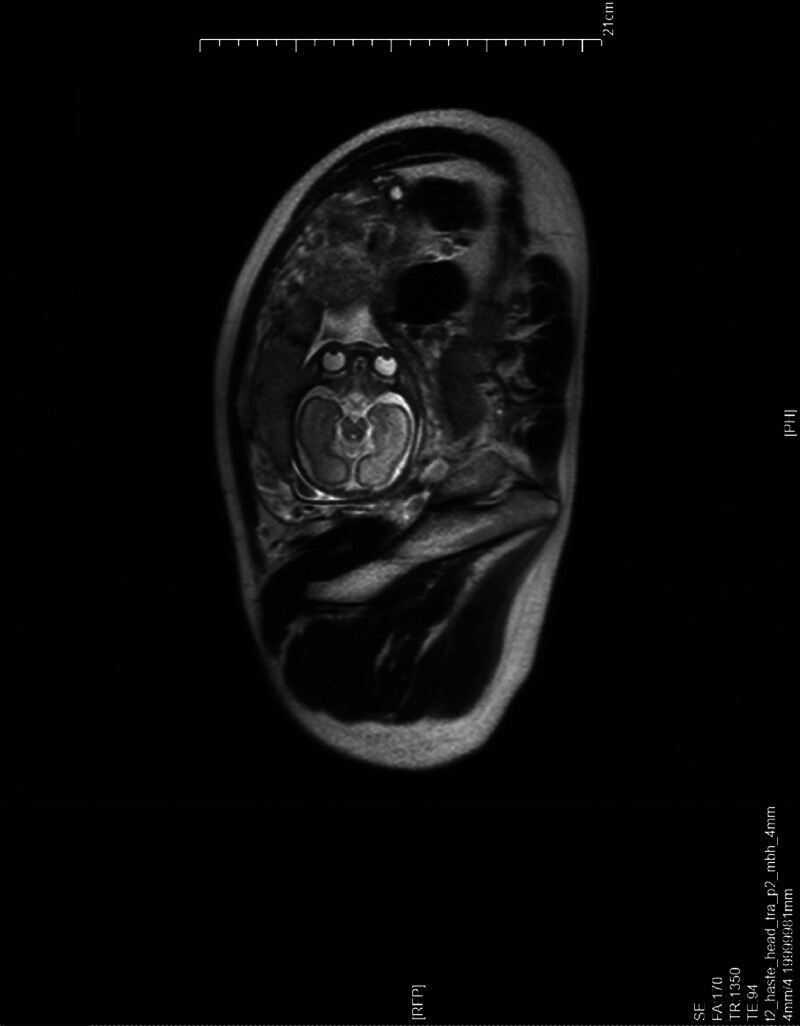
Fetal MRI at 27 weeks of gestation shows the subependymal cysts in the fetus. MRI, magnetic resonance imaging.
